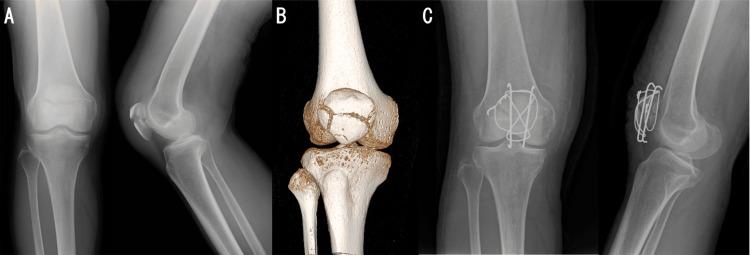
Imaging findings of the initial injury and primary surgery A: Preoperative plain radiographs (anteroposterior (AP) and lateral views) showing a four-part patellar fracture. B: Preoperative 3D computed tomography reconstruction highlighting the multi-fragmentary fracture pattern. C: Postoperative radiographs (AP and lateral views) after the primary ORIF using the CCW technique with additional circumferential wiring. CCW, cerclage compression wiring; ORIF, open reduction and internal fixation.
